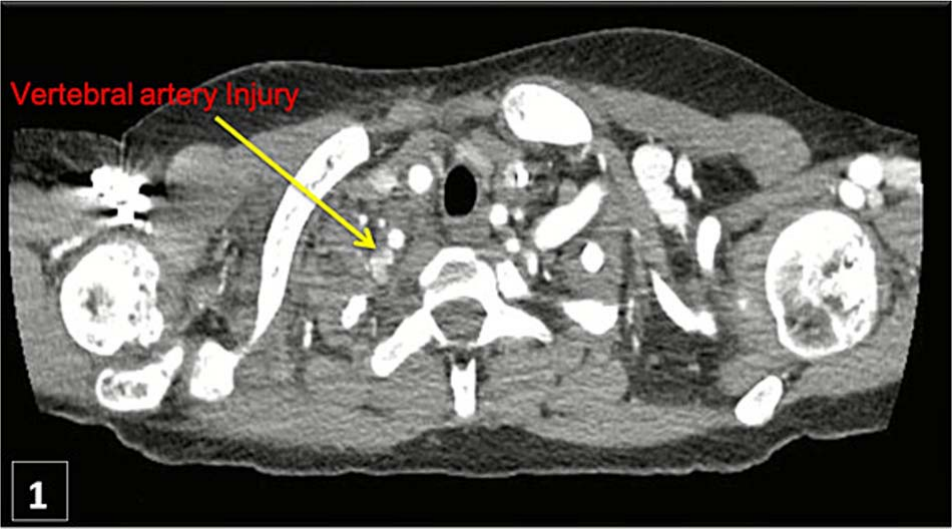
Thoracic CT angiogram shows contrast extravasation from left vertebral artery after CVC.
